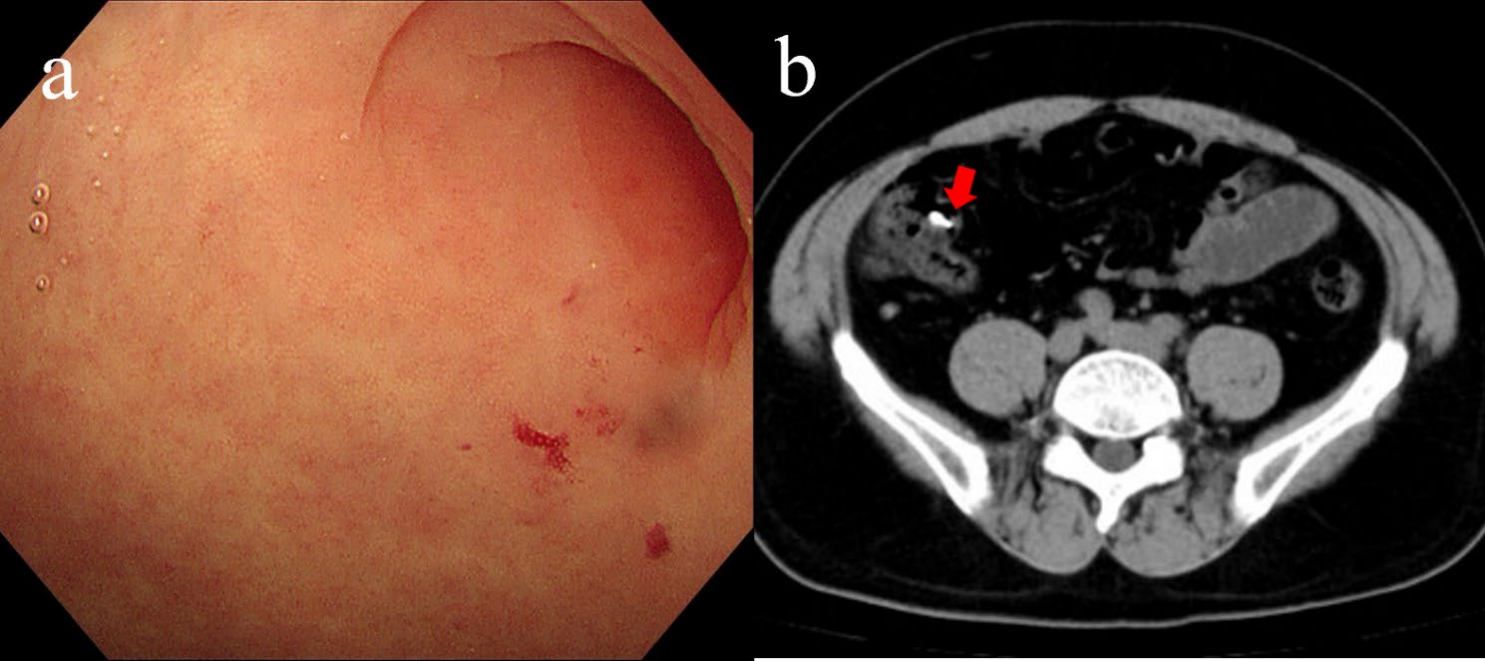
Appendiceal bleeding of unknown reason Case 1: (a) Bleeding was found at the appendix orifice after flushing and waiting. (b) Fecaloma (red arrow) was showed in contrast-enhanced CT
